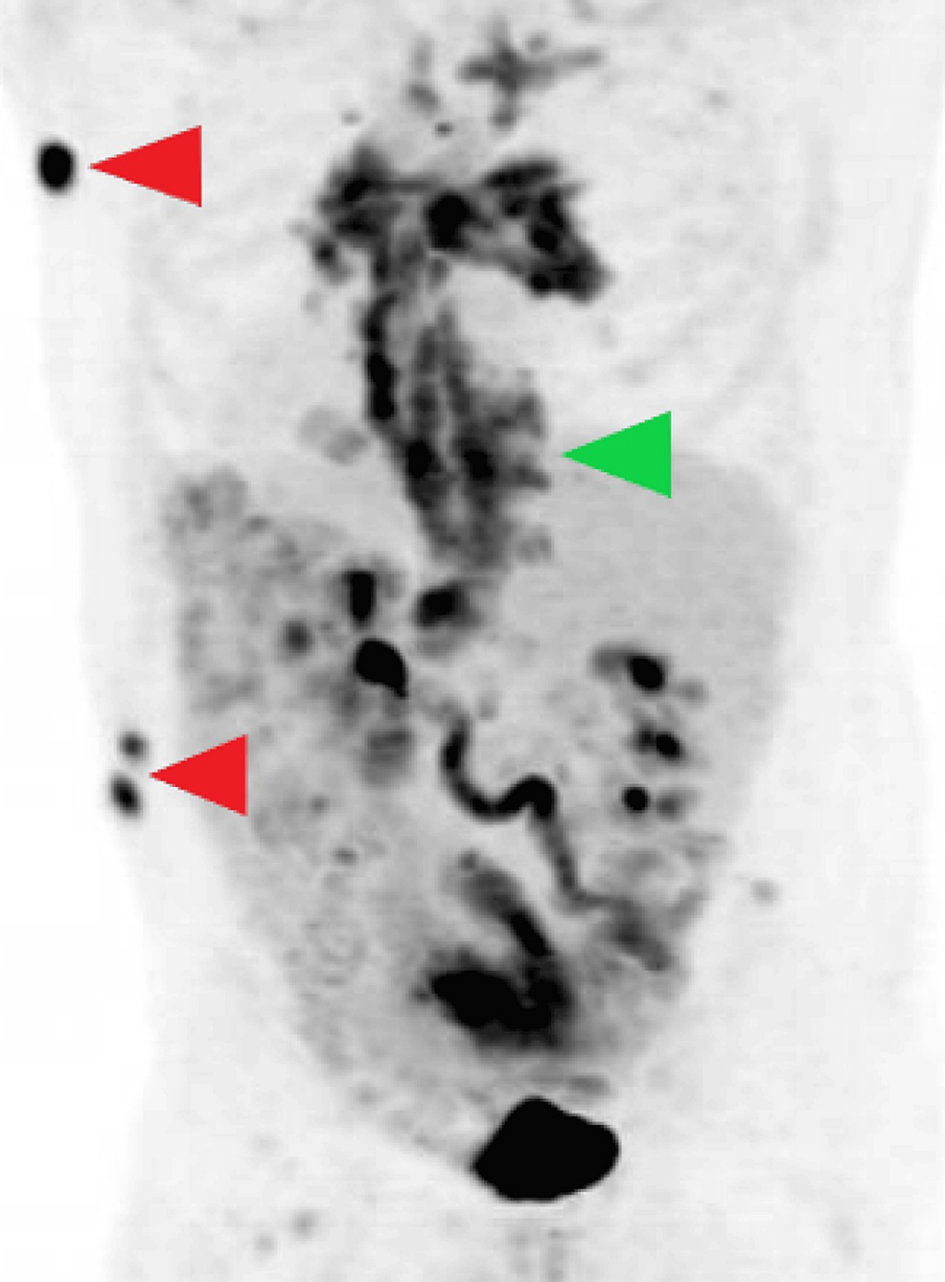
Fludeoxyglucose-18 (FDG) positron emission tomography (PET) scan. The green arrow points to FDG uptake associated with lytic destruction of T3, which has an SUV max of 8.9. Uptake extends into the spinal canal between T2 and T4. The red arrows point to multiple FDG-avid subcutaneous nodules. A 3 cm subcutaneous nodule inferiorly in the left axilla has an SUV max of 16.5. Two nodules in the left flank have an SUV max of 12.4. SUV max: maximum standardized uptake value.
